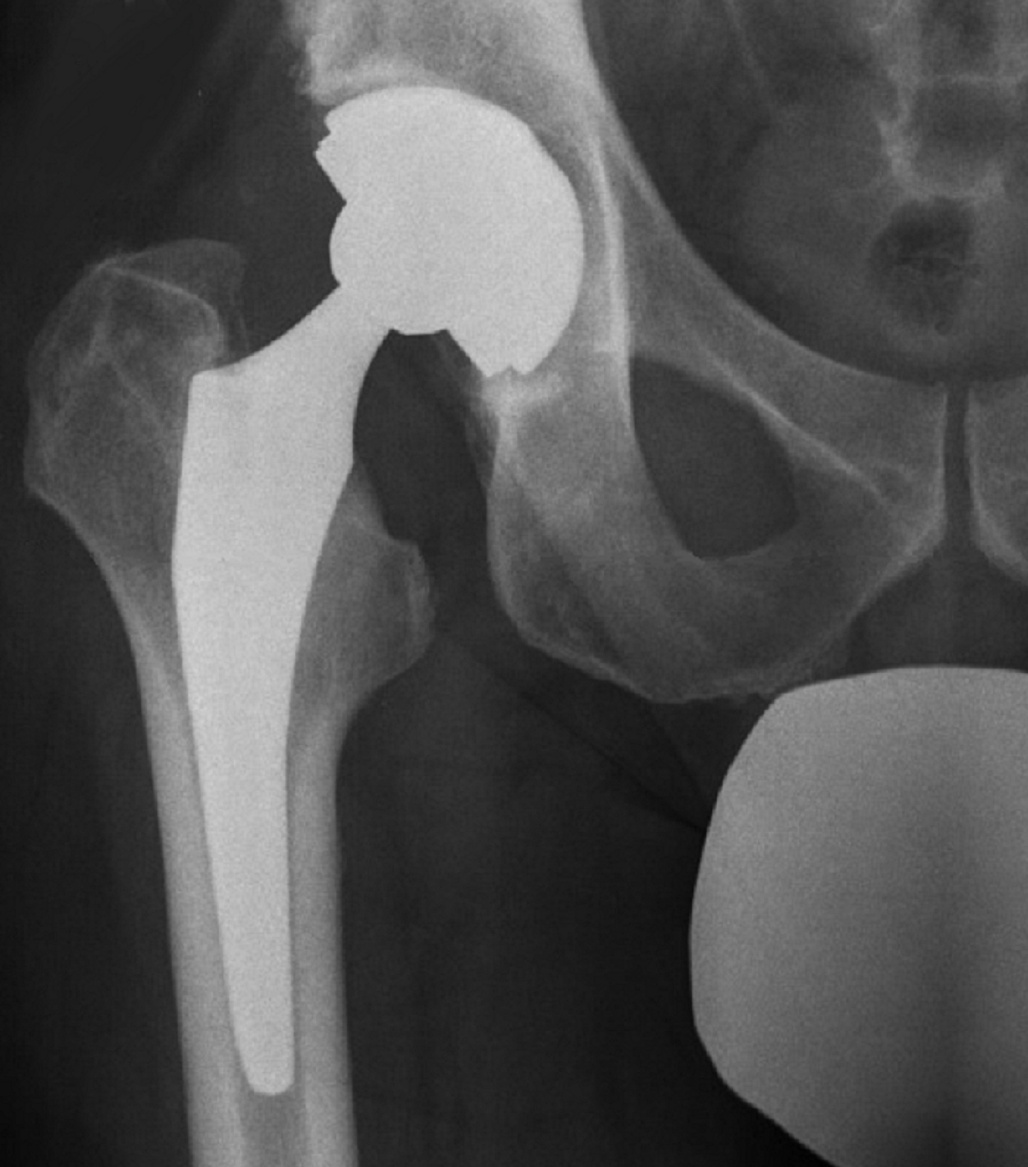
Typical radiographic appearance of IPI on AP pelvic film with insufficient anteversion of the acetabular component. Note the absence of radiographic ellipse at the cup opening. IPI, iliopsoas impingement; AP, anteroposterior
